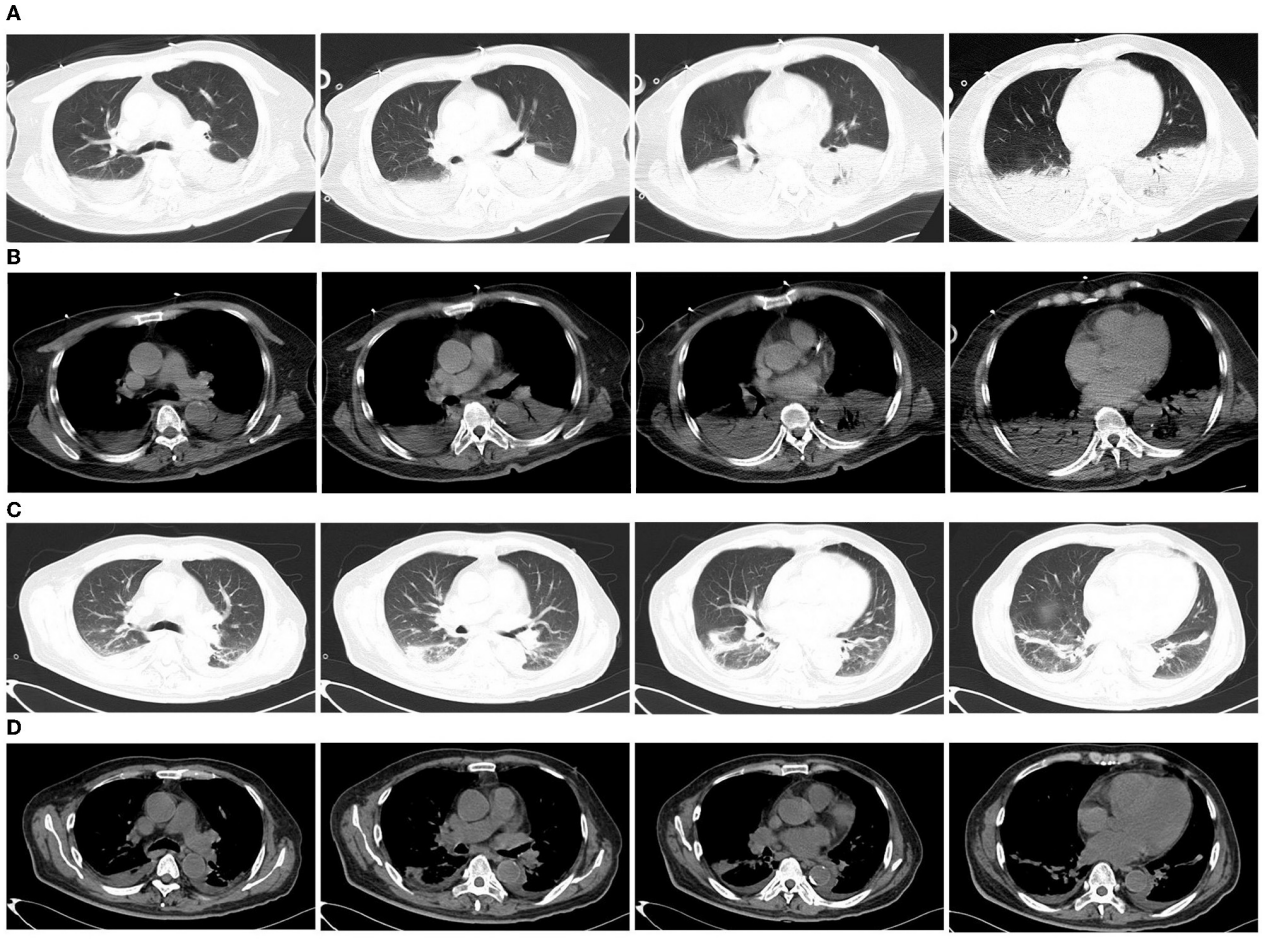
Chest computed tomography (CT) of the patient. (A, B) Chest CT on the first day of admission to the emergency department showed consolidation of the lower lobes of both lungs; (C, D) Chest CT 23 days after moxifloxacin treatment showed that the lesions were significantly absorbed.
